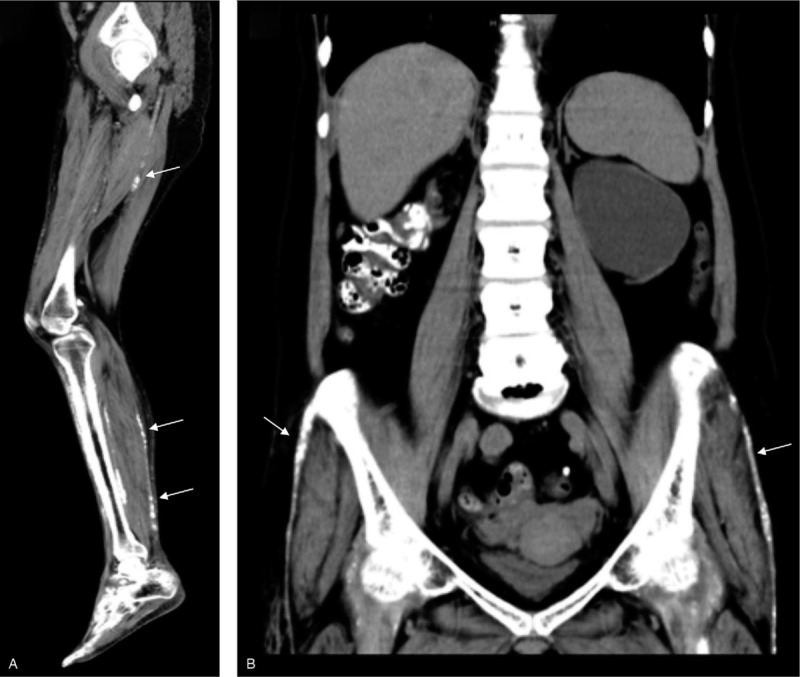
Computed tomography images of lower extremities. The images show calcification of the fascia in the lower extremities (A and B, white arrows).
